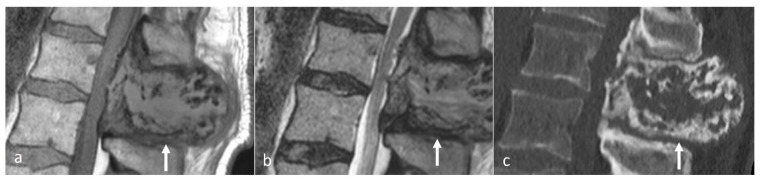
Sagittal T1 (a), T2 (b) and CT (c) images of a sclerotic myelomatous deposit involving a thoracic spinous process (white arrows). While myeloma typically tends to present as lytic lesions, occasionally they can be expansile and sclerotic as seen here.
